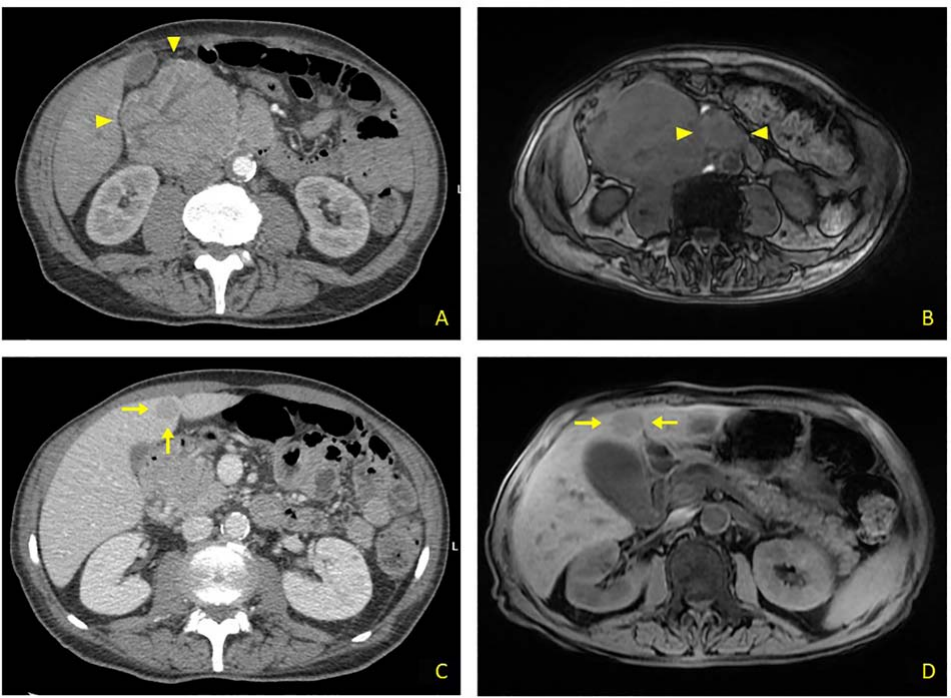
(A) Contrast CT scan: bulky formation with inhomogeneous uptake, arising from upper duodenal-pancreatic angle (arrowheads). (B) MRI: main lesion appears hypointense on fat-suppressed T1-weighted sequences. Evidence for further retromesenteric contrast enhanced tissue (arrowheads). (C) Contrast CT scan: hypodense rounded liver metastasis arising from segment V, measuring 2.5 cm in diameter (arrows). (D) MRI: T1-appearance of subglissonian pericholecystic liver metastasis (arrows).
